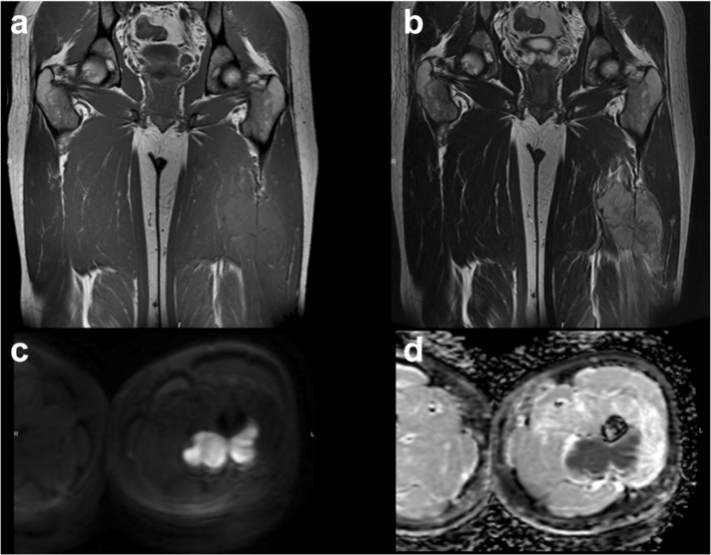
Thirty nine years old girl with a primitive neuroectodermal tumour (PNET). Coronal T1 weighted image (a), Coronal T2 weighted image (b), DWI B1000 (c-d)
